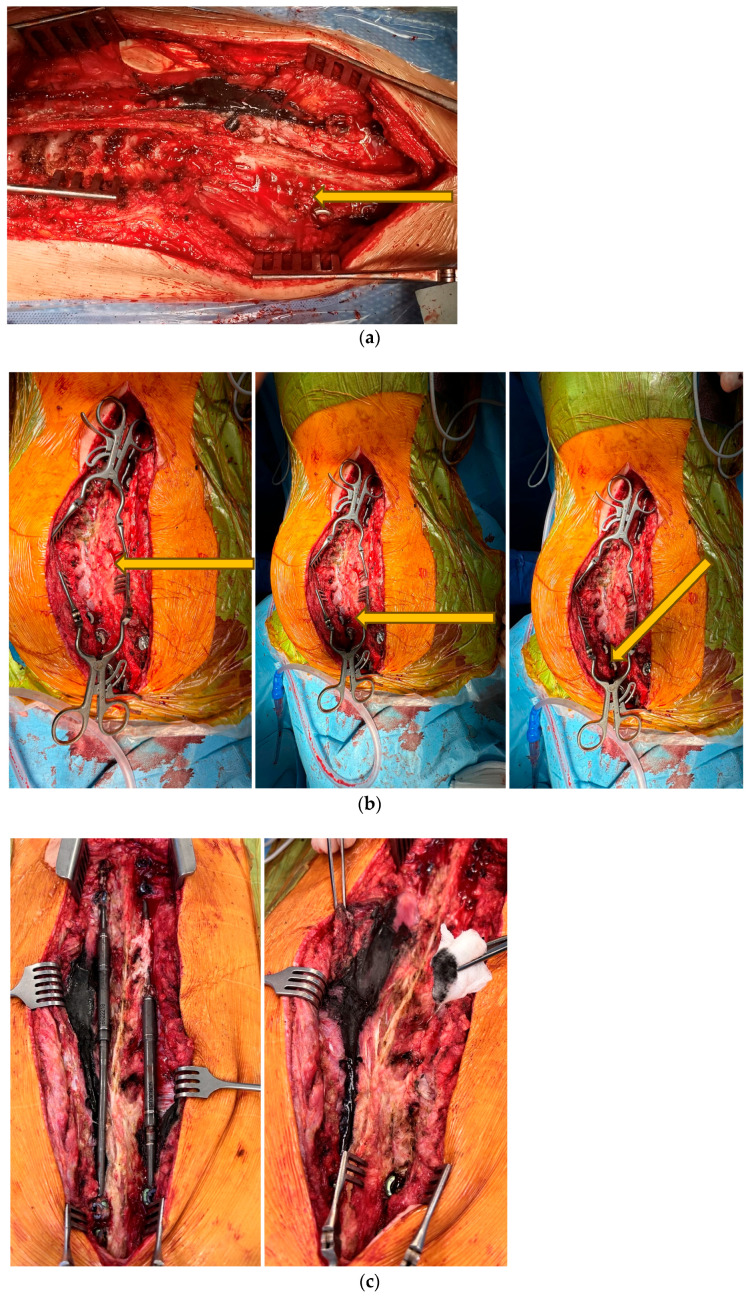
(a) X-rays and intraoperative pictures of 8-year-old girl with EOS treated with MCGRs, no complications observed during the 5-years follow-up and lengthening period, but spontaneous fusion, apex ribs fusion, and metallosis observed on the convex side during conversion to PSF, as the arrows show. However, apex ribs fusion is not always particularly visible, so we rely on observations and examination of the correction of the peak of the curve intraoperatively, after removal of both MCGRs. (b) Intraoperative pictures of 9-year-old girl with EOS treated with MCGRs, no complications observed during follow-up and lengthening period, but spontaneous fusion, apex ribs fusion, and metallosis observed on the convex side during conversion to PSF, as the arrows show. (c) Intraoperative pictures of 12-year-old girl with EOS treated with MCGRs, no complications observed during the follow-up and lengthening period, but spontaneous fusion, apex ribs fusion, and metallosis observed on the convex side during conversion to PSF at 3-years of follow-up period.
